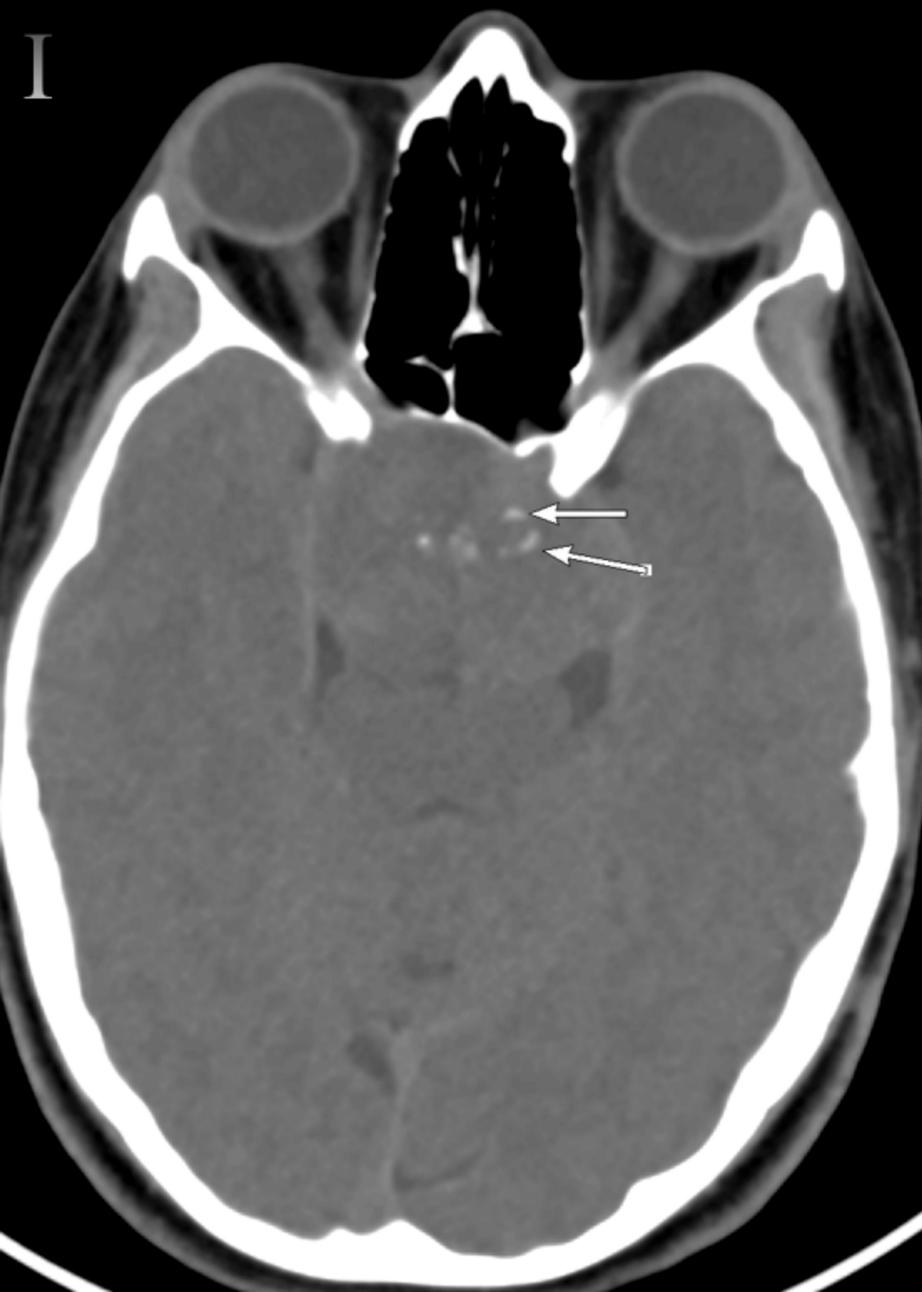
Soft window axial CT (I) shows several flecks of calcification (long arrows) CT: computed tomography
